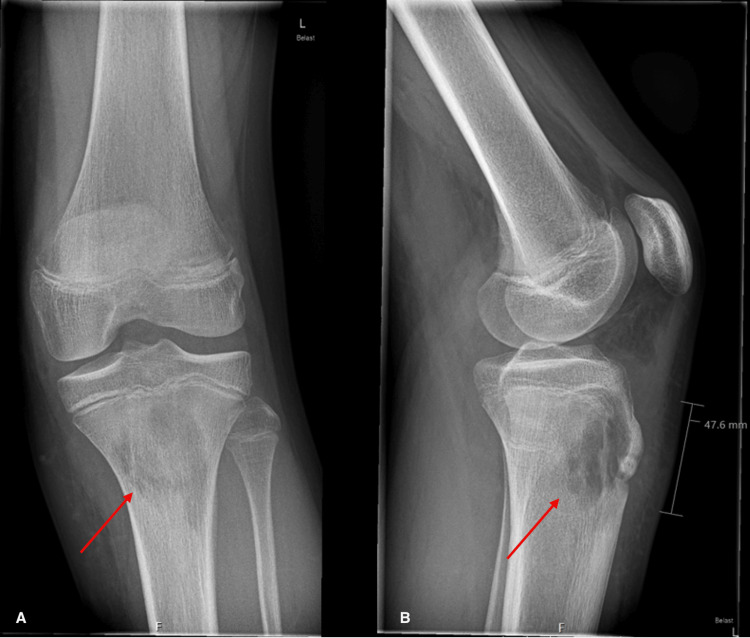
Plain radiograph Plain radiograph of the left knee demonstrating a lytic lesion (red arrow) in the proximal tibial metaphysis with ill-defined margins, suspicious for underlying bone pathology.
